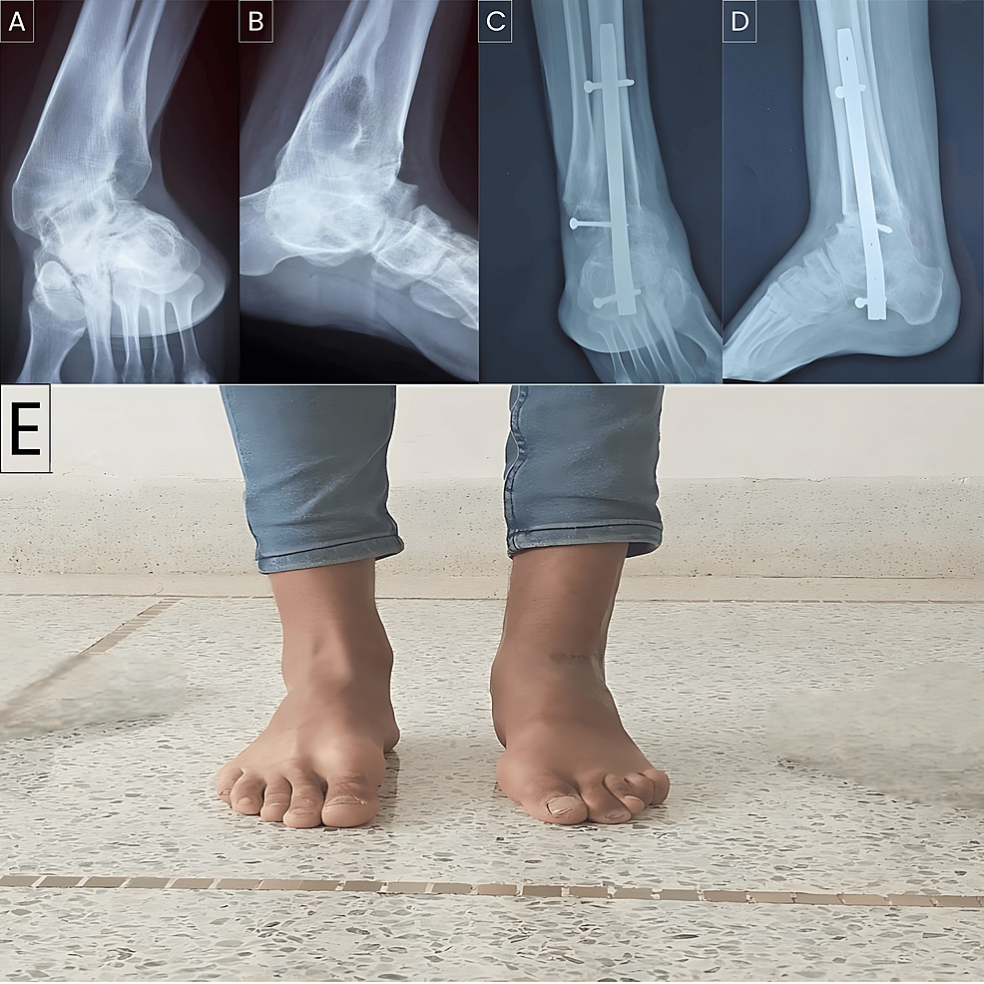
Charcot's disease is complicated by the sequelae of septic arthritis. Anteroposterior (A) and lateral (B) X-ray images of the ankle show malunion of the ankle and a geode in the distal quarter of the tibia. Anteroposterior (C) and lateral (D) X-ray images of Tibio-talo-calcaneal arthrodesis with retrograde femoral nail: results nine months postoperatively. E: Functional outcome nine months after tibio-talo-calcaneal arthrodesis
